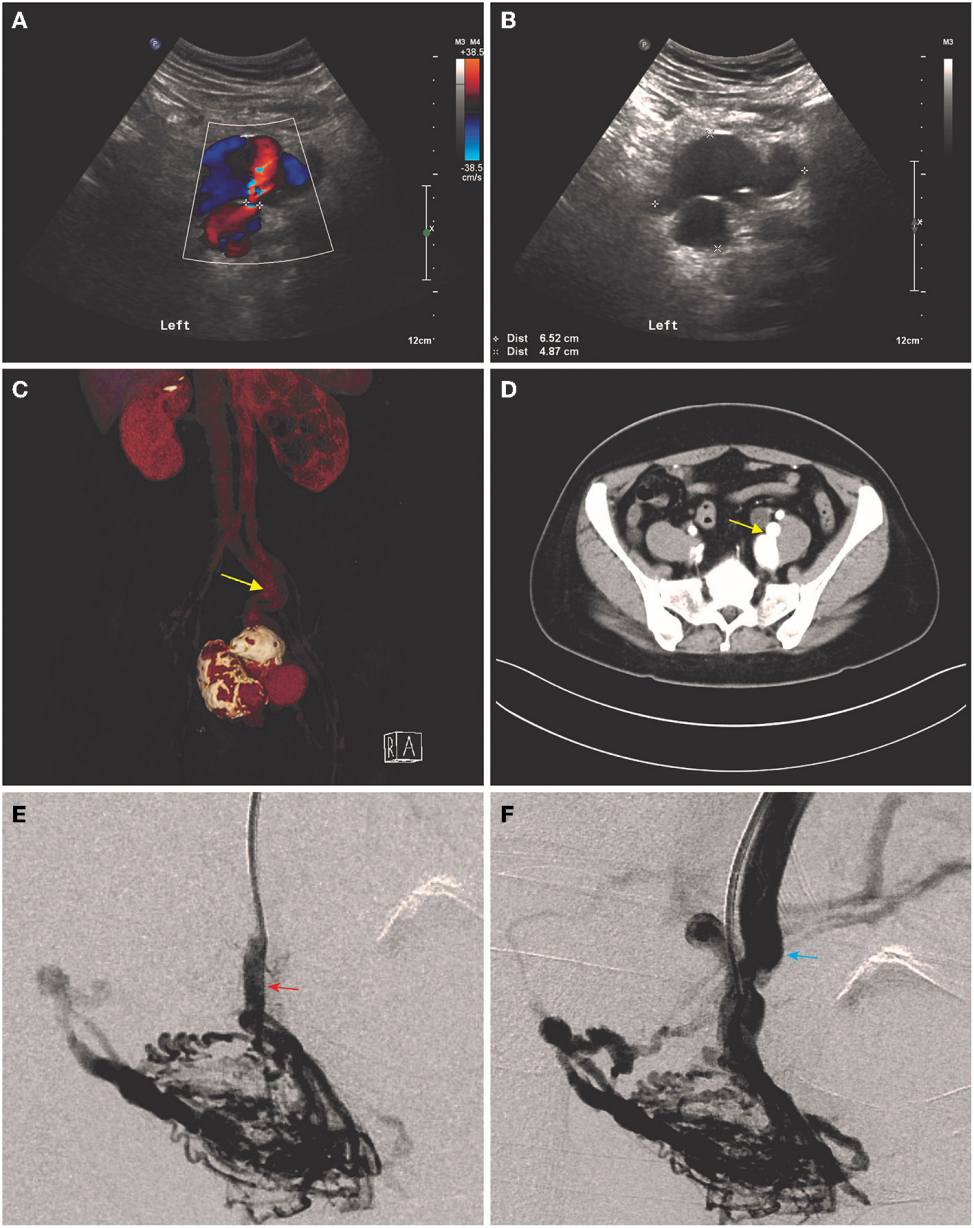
Typical imaging presentations of iliac arteriovenous fistulas (IAVFs). (A) Multicolored blood flow can be seen in the proximal artery during both the systole and diastole in ultrasound. (B) Aneurysm and venous aneurysm formation were shown in ultrasound. (C,D) CT angiography (CTA) and cross-sectional image showed a direct connection (yellow arrows) between internal left iliac artery and vein accompanied with aneurysm and venous aneurysm formation. (E,F). Digital subtraction angiography (DSA) showed multiple connections between a branch of inflow left iliac artery (red arrow) and outflow left internal iliac vein.
